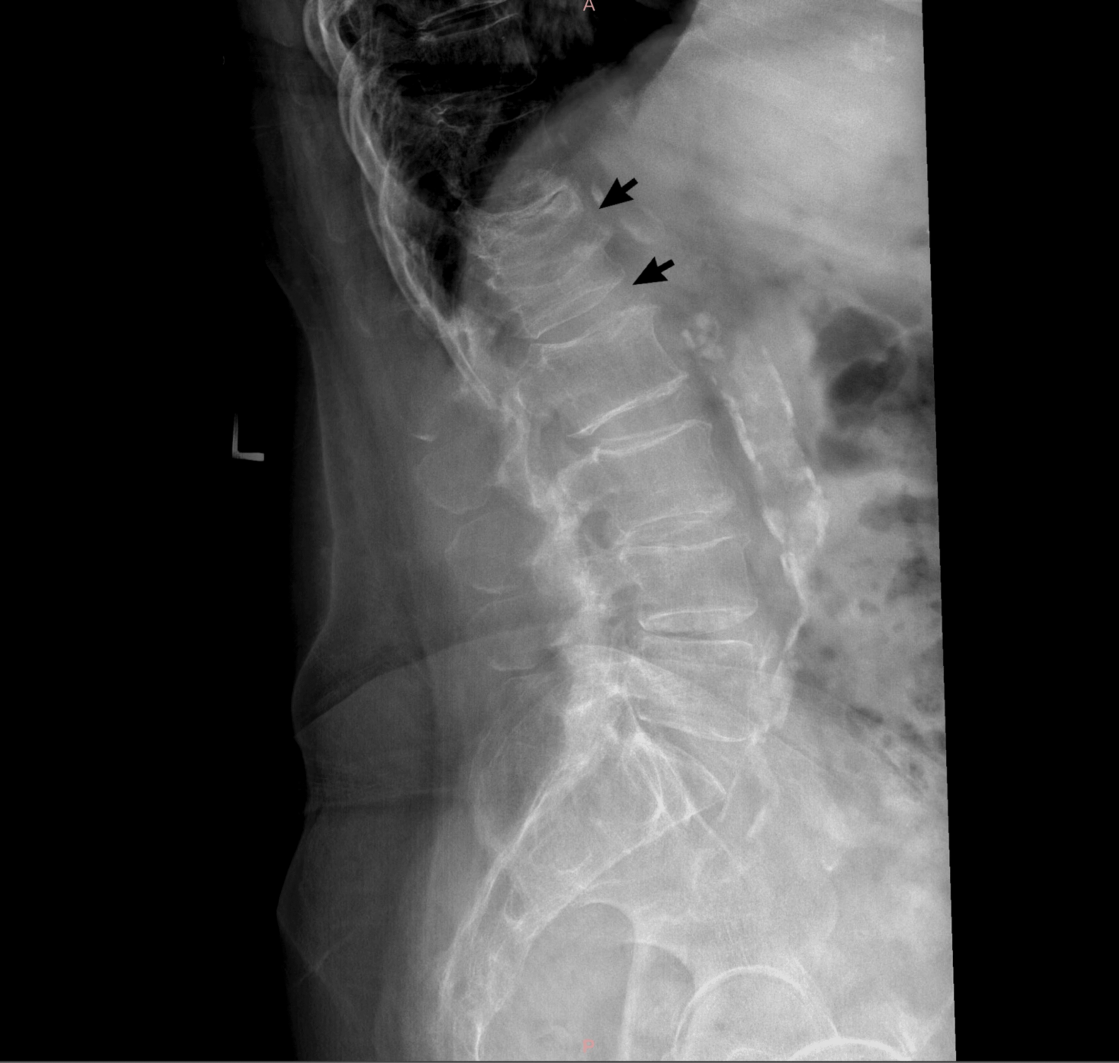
X-ray imaging showcasing degenerative scoliosis and stenosis at L3-L4 level, as well as an L1 fracture
